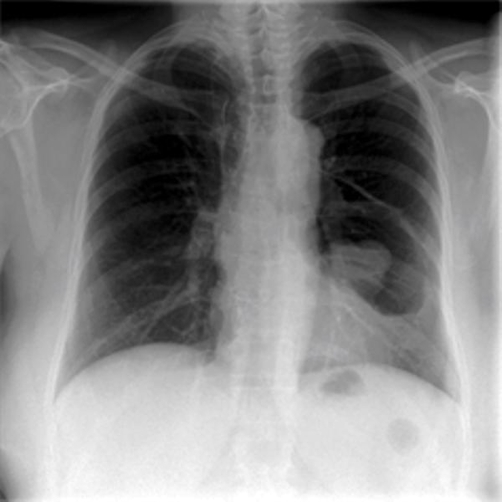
Chest radiograph demonstrating a mass in the left middle field.
